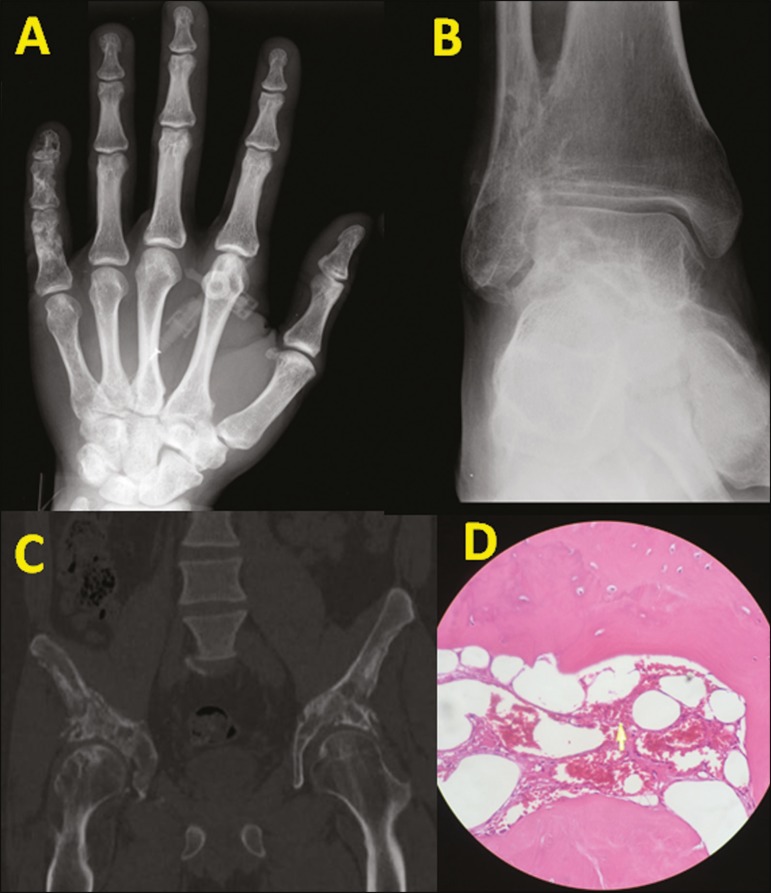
A: X-ray of the right hand, demonstrating permeative osteolytic
